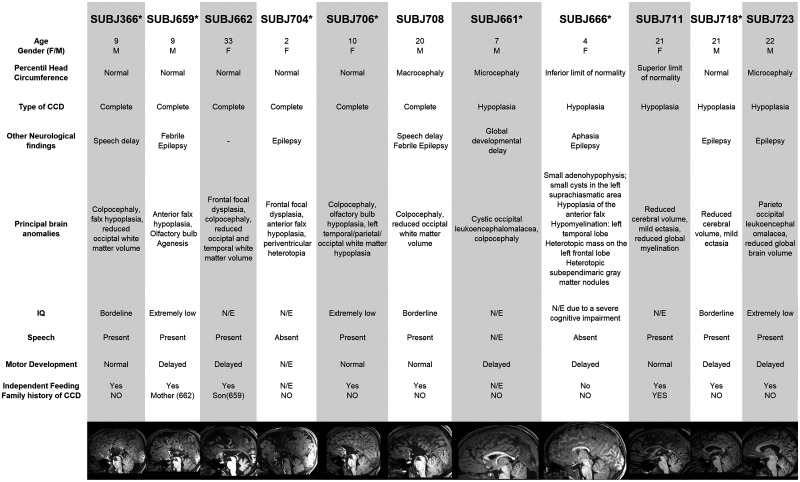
Clinical/radiological demographic summary of the CCD patients. Patients’ codes are noted on top. Asterisks denote that the patient was sedated during the MRI sessions. The bottom row shows a sagittal T1-weighted MRI of the patients’ brains. N/E, not examined.
